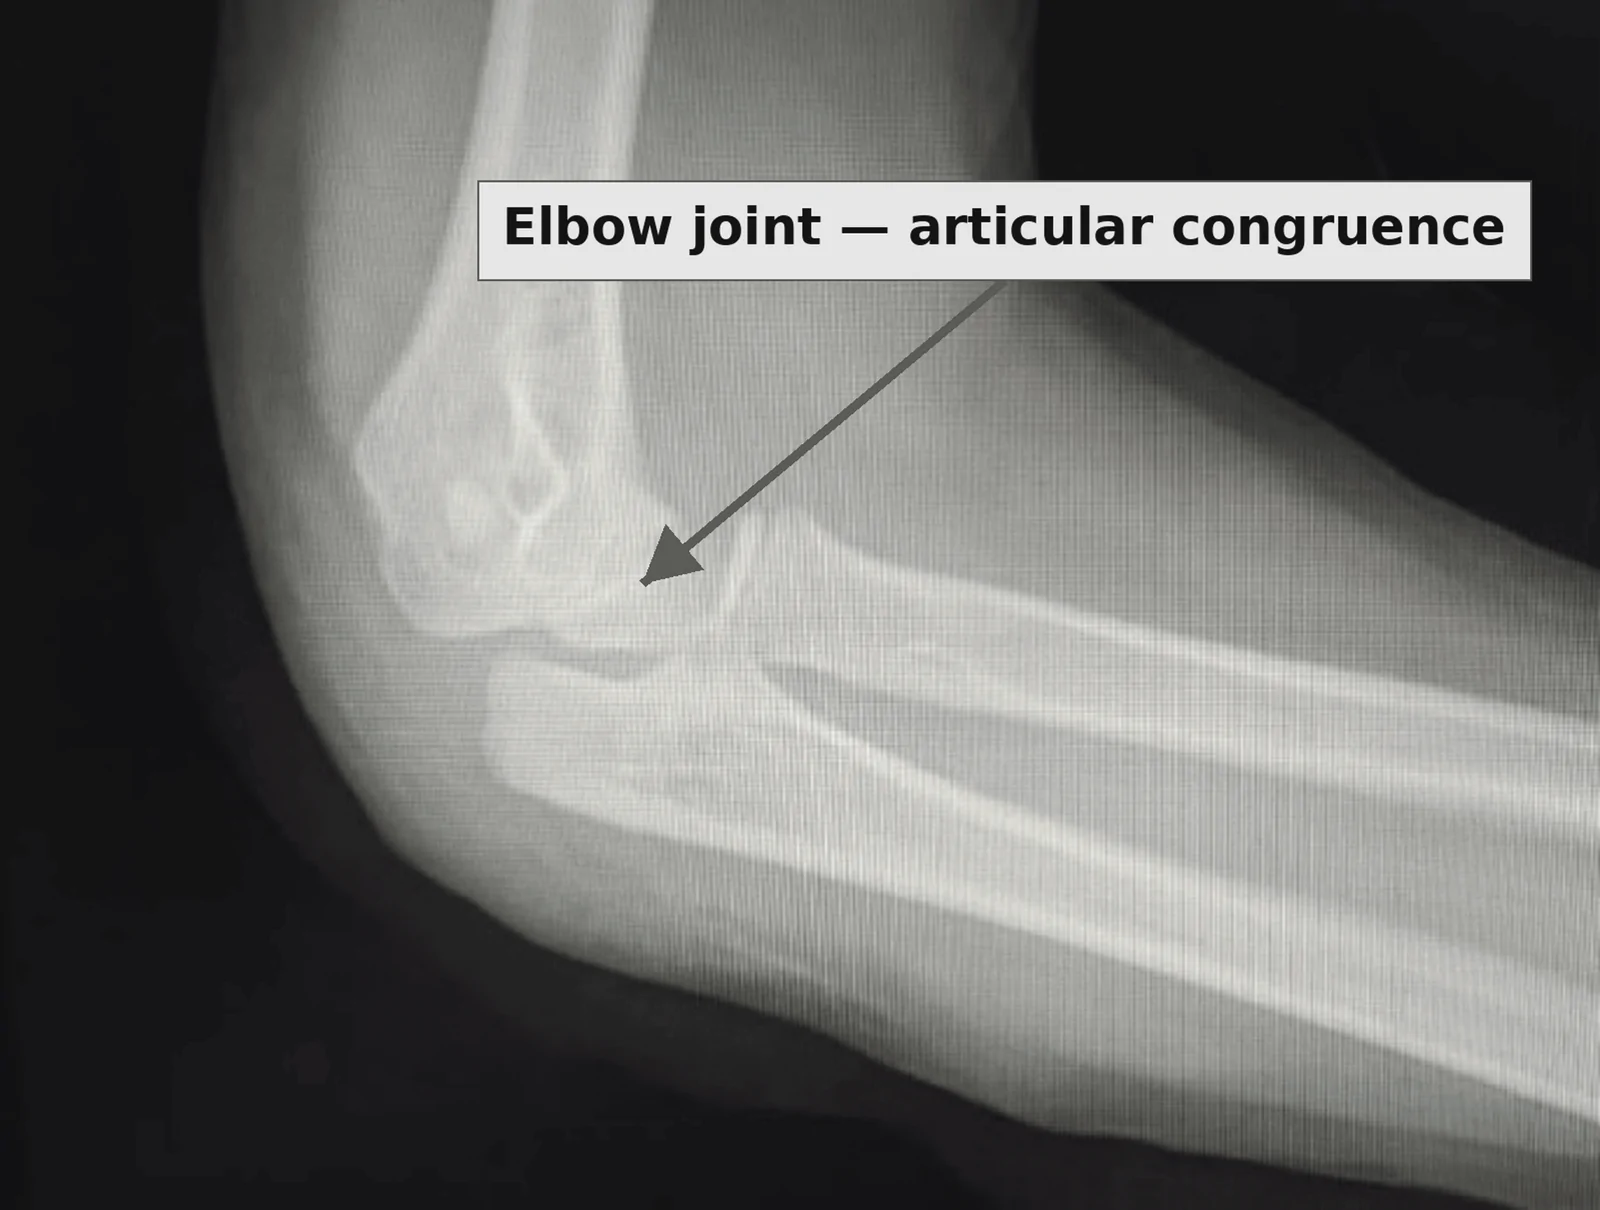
Lateral radiograph of the right elbow following closed reduction demonstrating satisfactory restoration of articular congruence between the proximal ulna and the humeral condyle.
